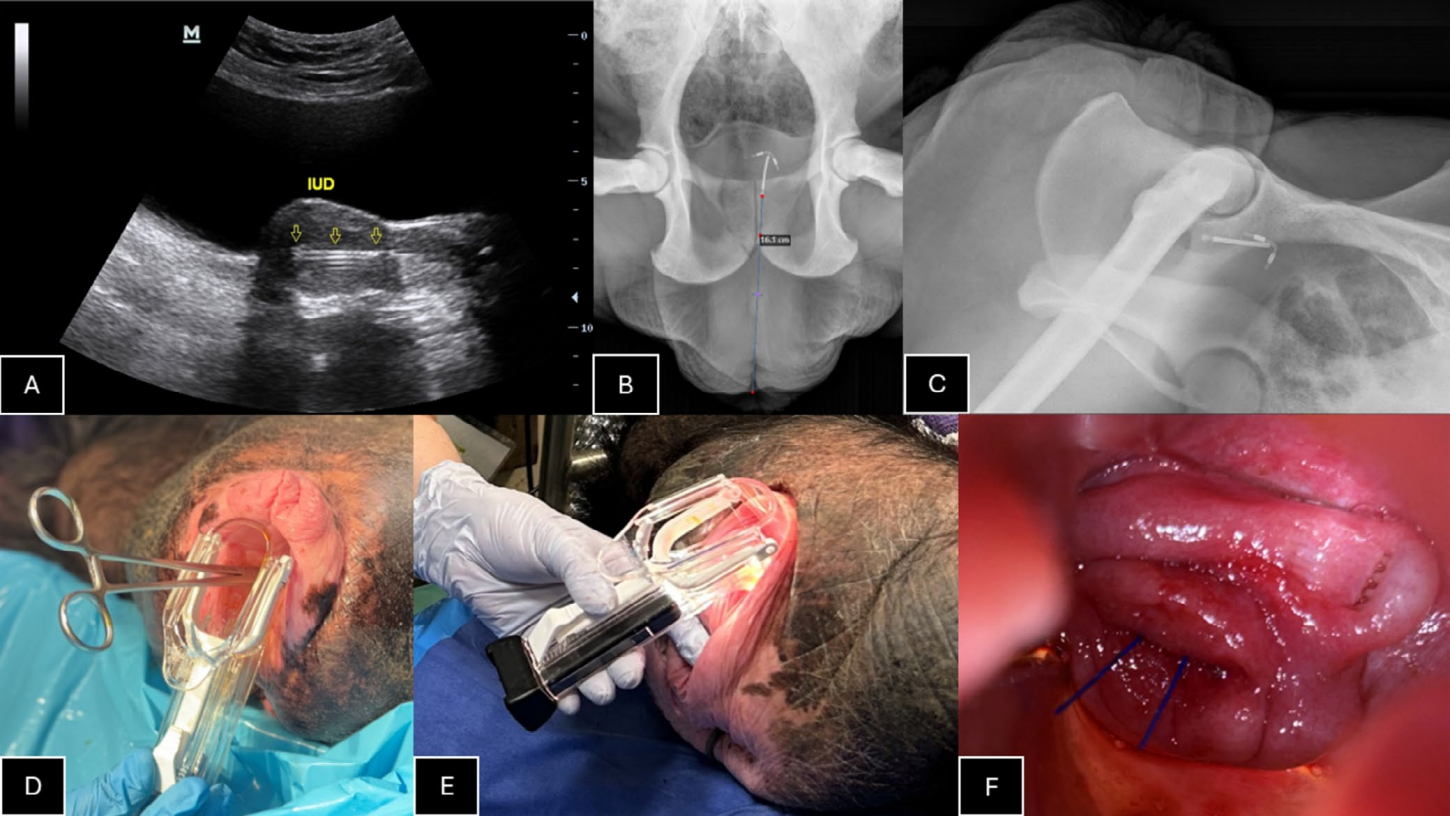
Ultrasonography showing IUD in uterus in AF1 (A), ventrodorsal and lateral pelvic radiograph in AF1 (B, C), disposable speculum in use without illumination in AF1 and with illumination in AF2 (D, E), cervical os with shortened strings of IUD visible in AF2 (F).
